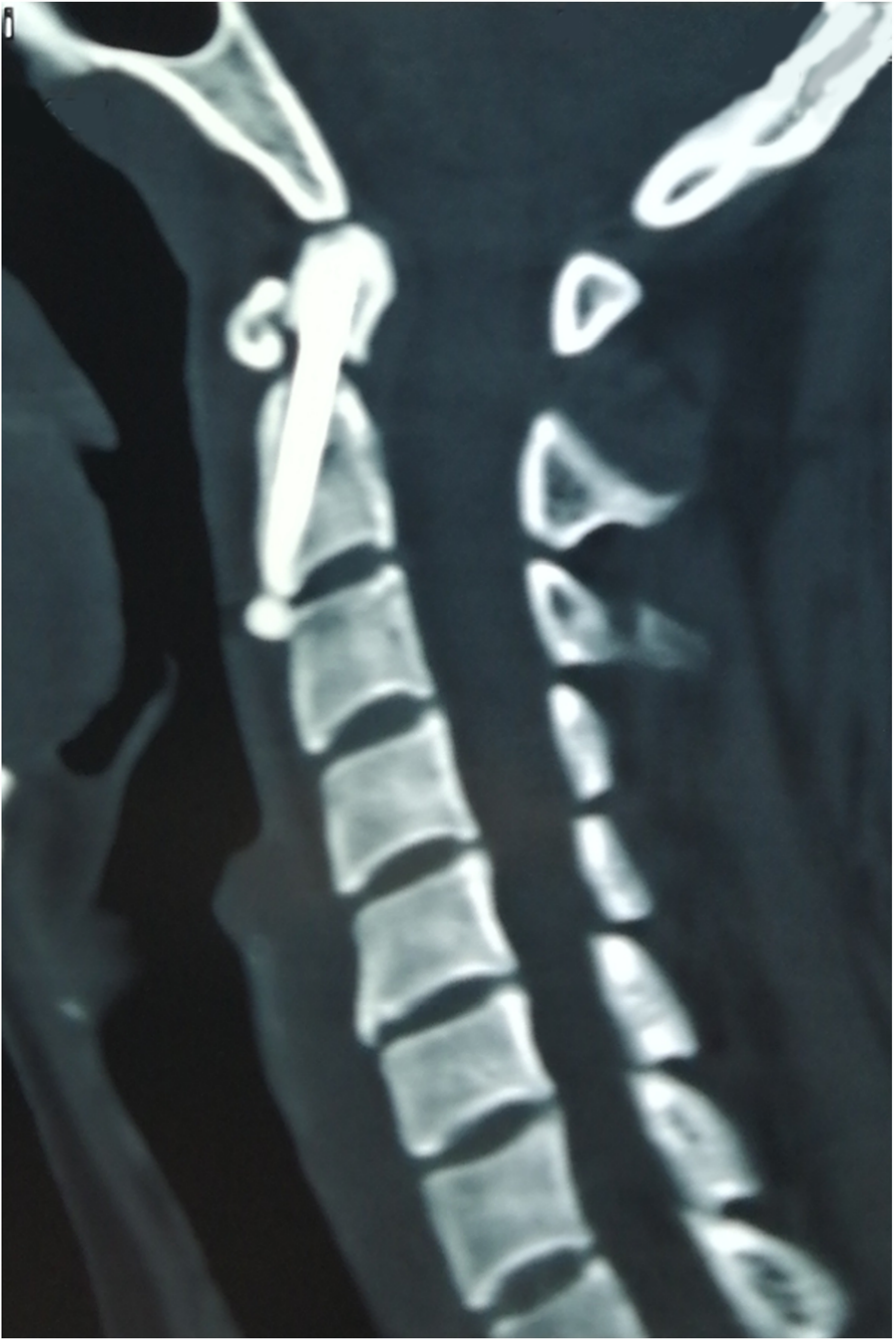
The CT scans showed screw fixation and nonunion of the odontoid fracture 6 months after surgery. Clear fracture line was found with osteosclerosis
